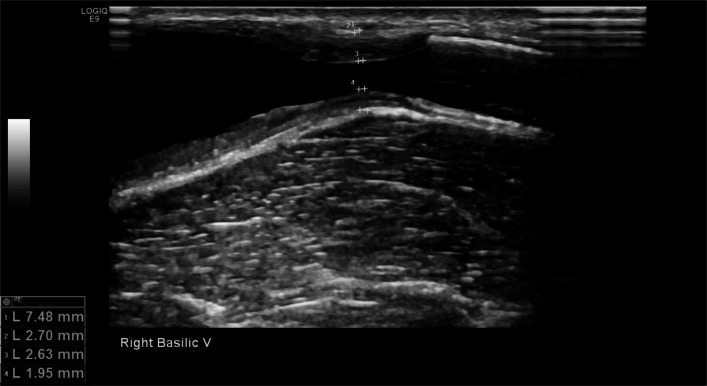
B-Mode ultrasound image demonstrating a basilic venous stenosis proximal to a stent graft. Measurements of outer-outer diameter, luminal diameter, near wall, and far wall IMT have been taken as shown
